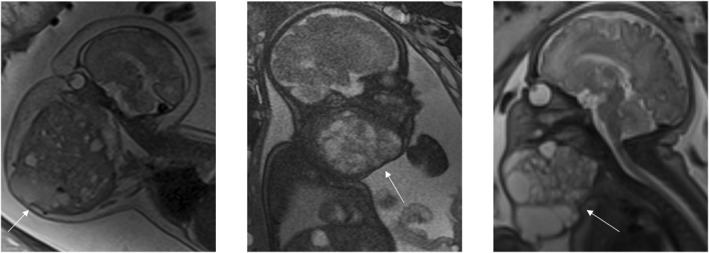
Fetal MRI images of teratoma. Left image ‐ T2 haste sagittal image of a 22 weeks fetus with an extensive neck and facial mass with solid and cystic components and airway displacement. Middle image ‐ FIESTA 60 sagittal imaging of fetus with a large cystic/solid mass on the left side of the neck, crossing over midline with Compression of the vessels and trachea. Right image ‐ T2 haste sagittal image of a 31 weeks fetus with an anterior cystic neck mass with some solid components.
